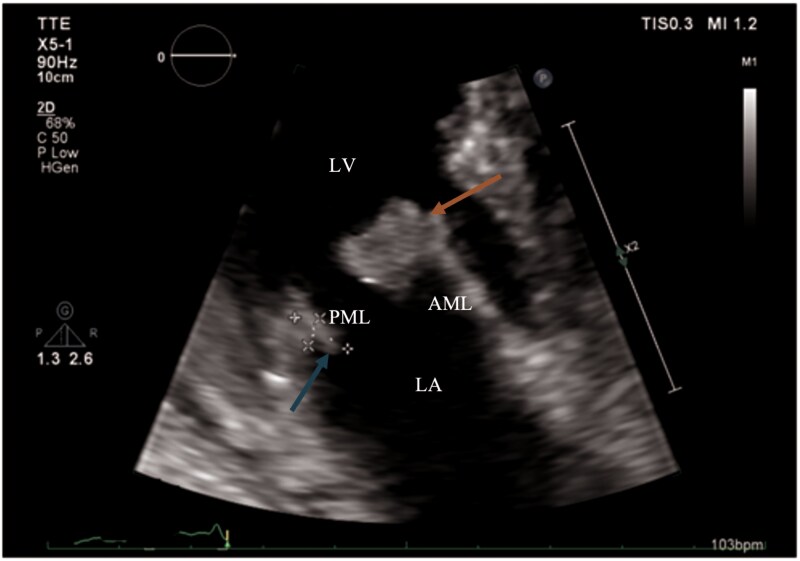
Transthoracic echocardiography (16 June 2023), three-chamber zoom view: mass on the anterior mitral leaflet (18× 15 mm, arrow) and new mass on the posterior mitral leaflet (8.5× 4.0 mm, arrow). Anatomical landmarks: AML, anterior mitral leaflet; PML, posterior mitral leaflet; LA, left atrium; LV, left ventricle.
